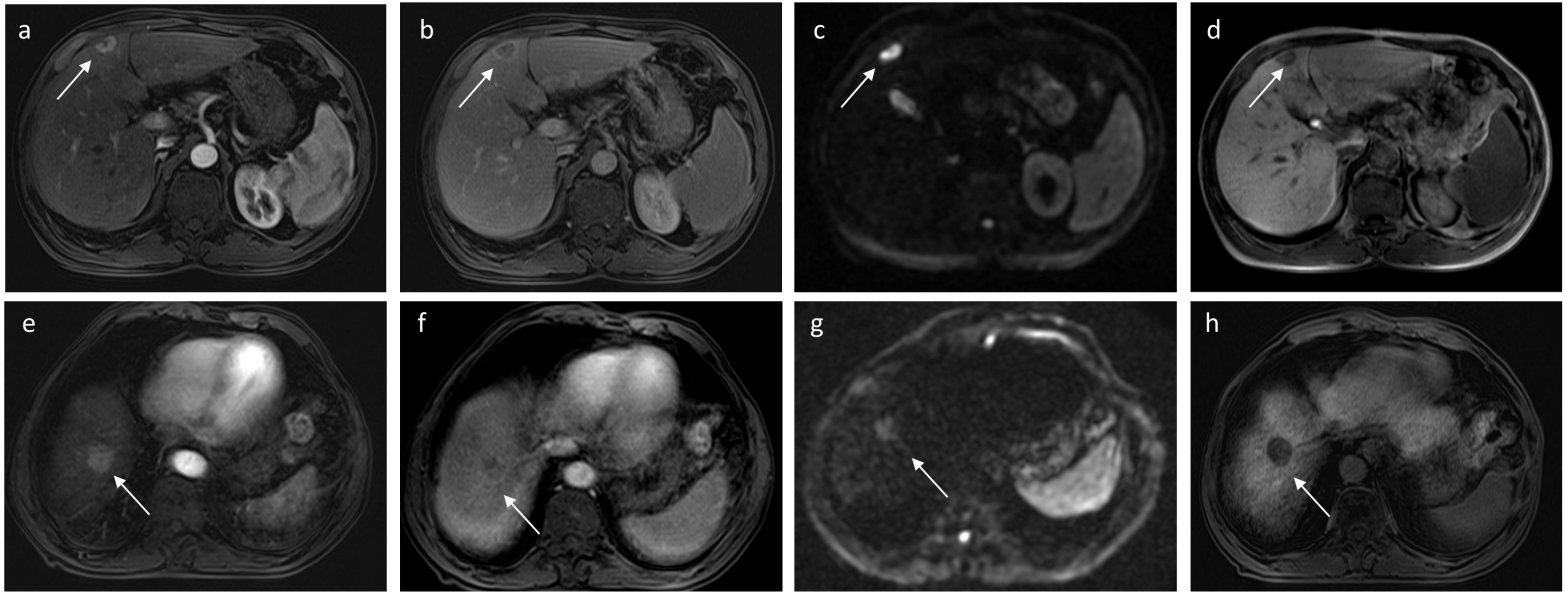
A 56-year-old man with sHCC was observed with early IDR after receiving RFA as the first-line therapy. Axial arterial (A) and delayed phases (B) show a well-defined tumor (white arrow) with rim APHE in hepatic segment IV. On the DWI (C), the tumor exhibits targetoid restriction and the tumors showed targetoid HBP appearance on hepatobiliary phase (D). Another 76-year-old man with HCC was observed with no recurrence after RFA during 13 months of the follow-up period. Axial arterial (E) and delayed phases (F) show a well-defined tumor (white arrow) with APHE in hepatic segment VIII. On the DWI (G), the tumor exhibits appeared restriction, and the tumors exhibit completely hypointense on hepatobiliary phase (H).
